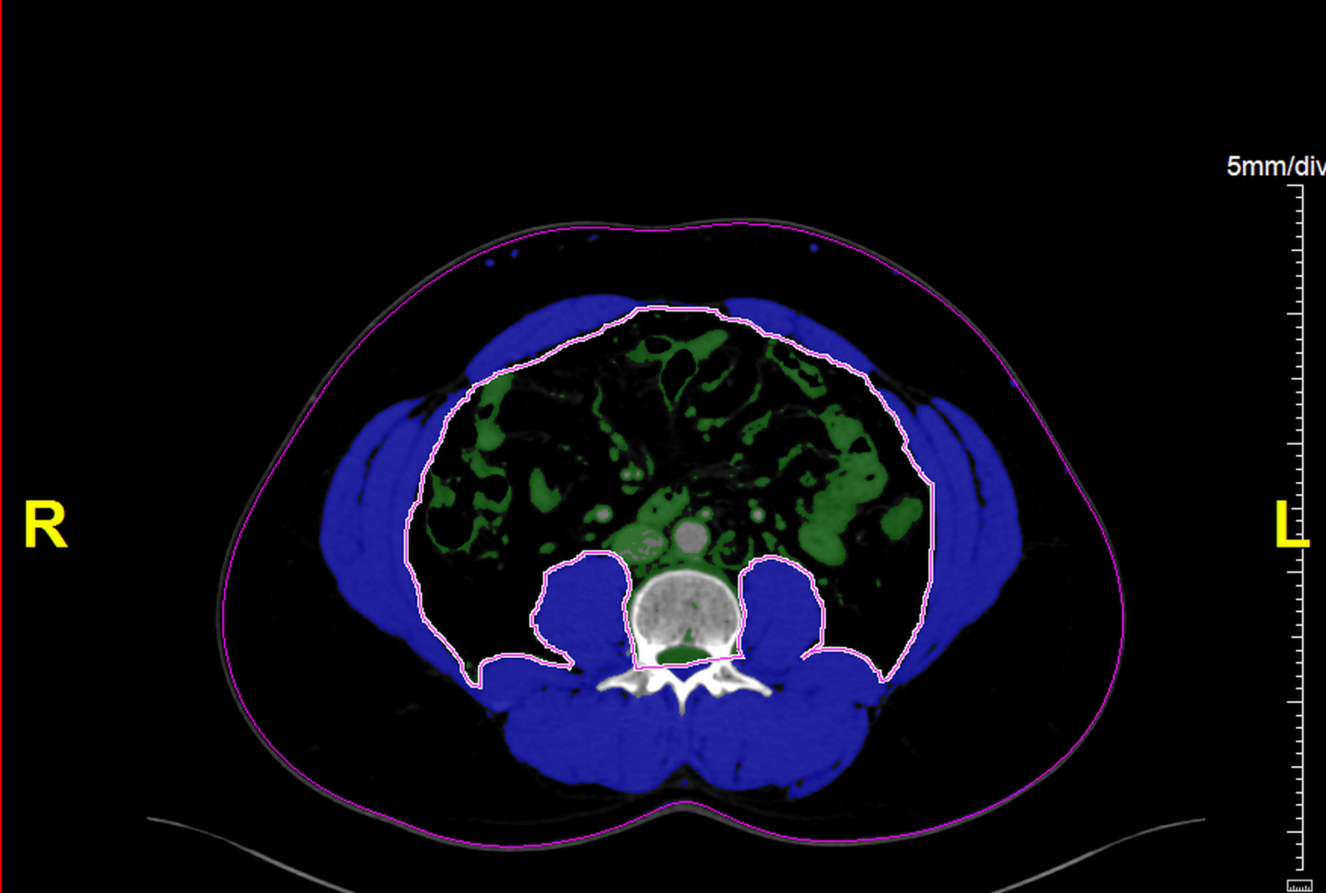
CT scan at the mid-L3 level for L3SMA assessment Skeletal muscle is highlighted in blue, with HU values set at -29 to +150 HU. In this example, L3SMA measures 155 cm². L3SMA, third lumbar vertebra skeletal muscle area
